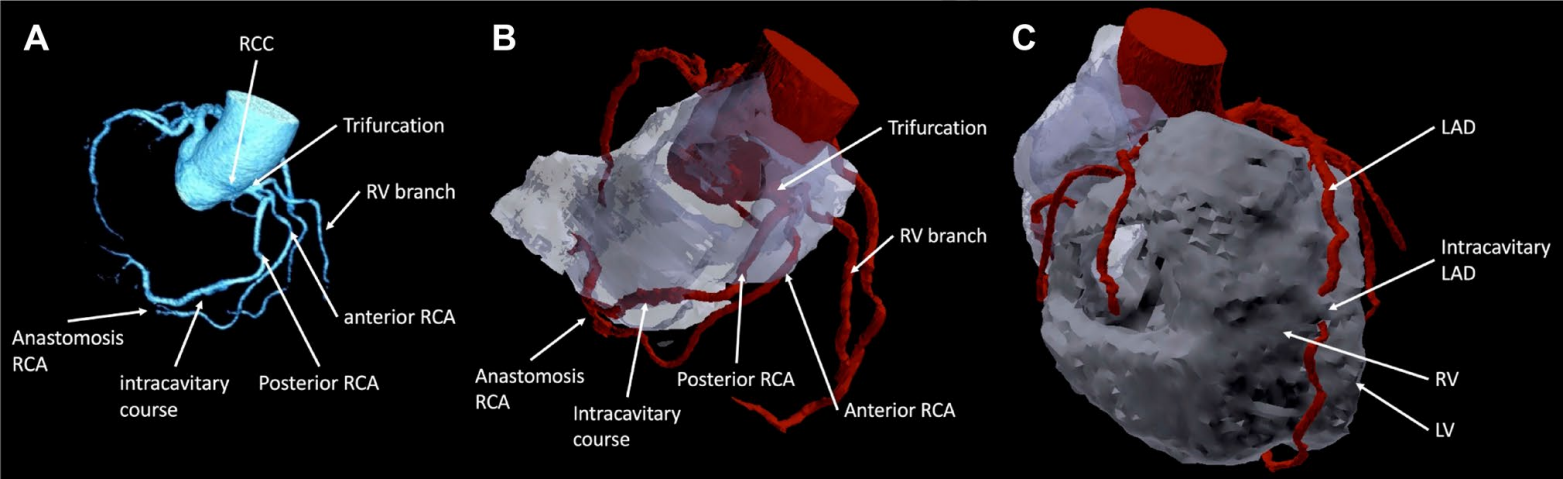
Multiple coronary anomalies. Panel A Multiplanar CTCA reconstruction of coronary tree showing four anomalies of the RCA (trifurcation, double RCA, intracavitary course, anastomosis). Panel B 3D-PDF model rendered from original CT data indicating the above mentioned RCA anomalies. The grey, transparent area represents the right atrium. Panel C 3D-PDF showing short intracavitary course of the mid LAD within the right ventricle. *CTCA* Computed tomography coronary angiography, *LAD* left anterior descending coronary artery, *LV* left ventricle, *RCA* right coronary artery, *RCC* right coronary cusp, *RV* right ventricle
